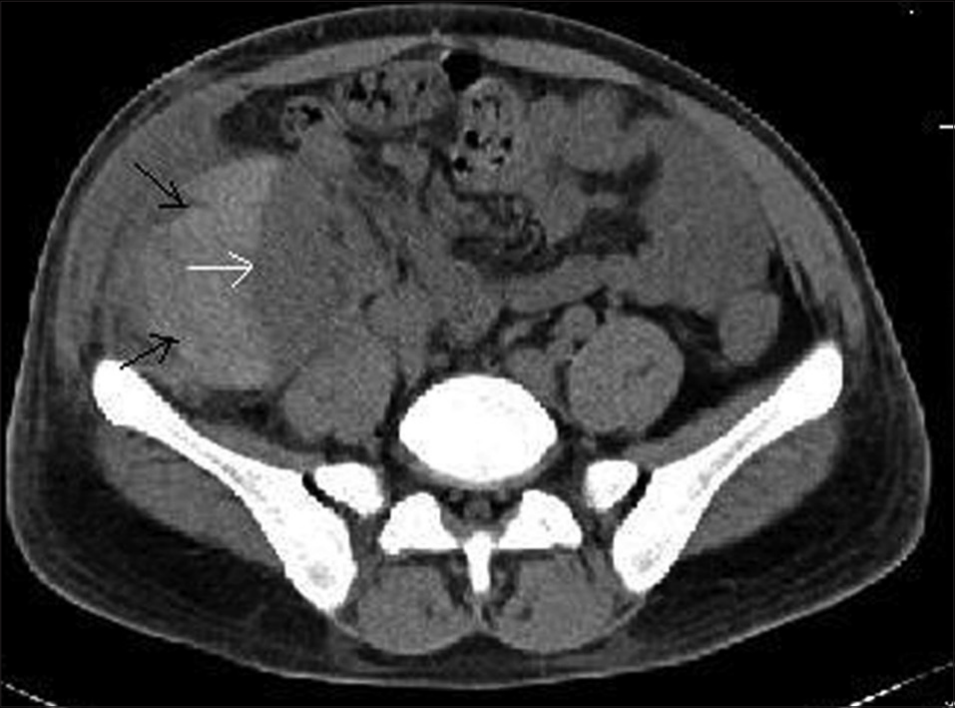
CT of the abdomen (transverse section) showing sub capsular hematoma (black arrows) compressing the graft kidney (white arrow)
